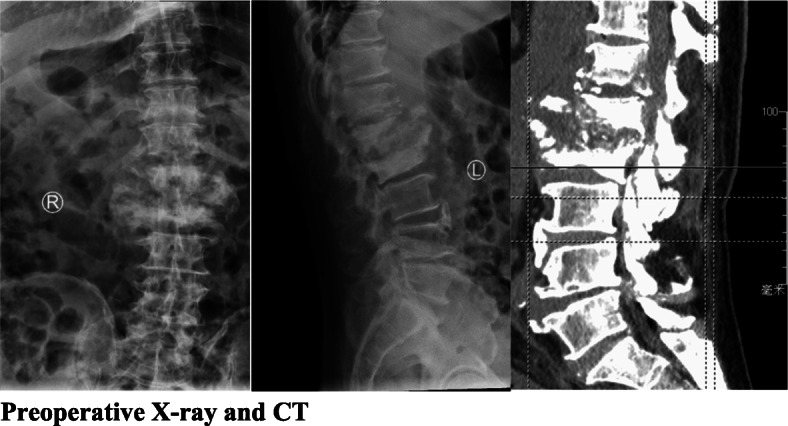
Preoperative X-ray and CT
